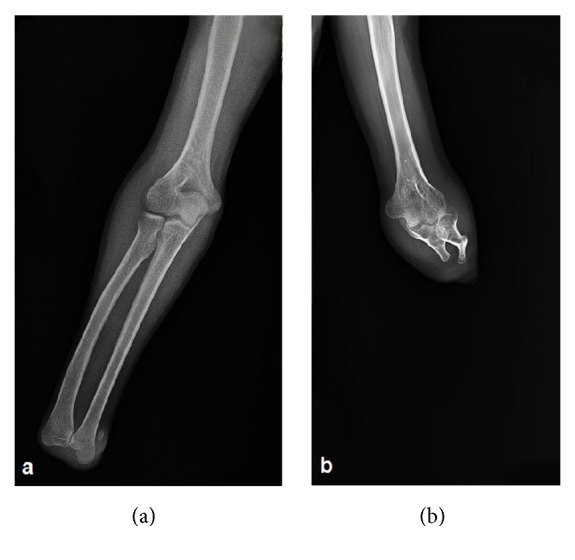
Anteroposterior radiographs of the right (a) and the left (b) arms demonstrating the absence of the right hand with well-developed radius and ulna, and the absence of the left forearm distal to the elbow where there are small radial and ulnar segments participating in the elbow joint. There is a rudimentary bone of about 1 cm in the medial neighborhood of the medial aspect of the right distal ulna.
